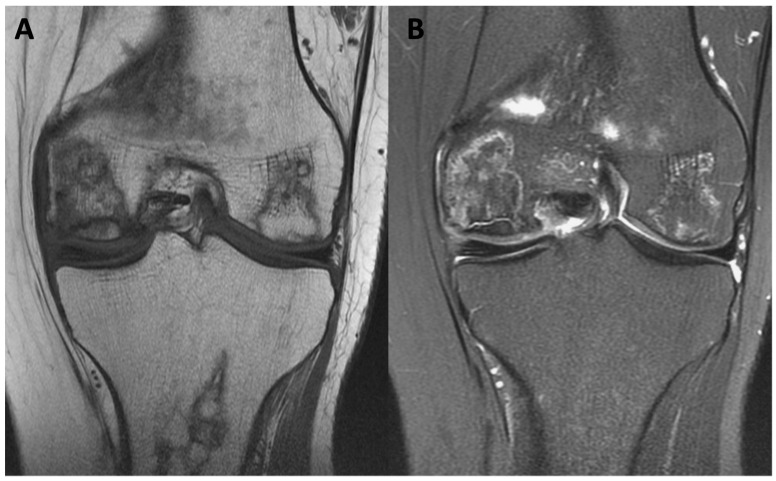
MRI image of AVN of the lateral and medial condyle of the femoral bone of the knee joint. (A) T1-weighted image; (B) short T1 inversion recovery (STIR) image.
